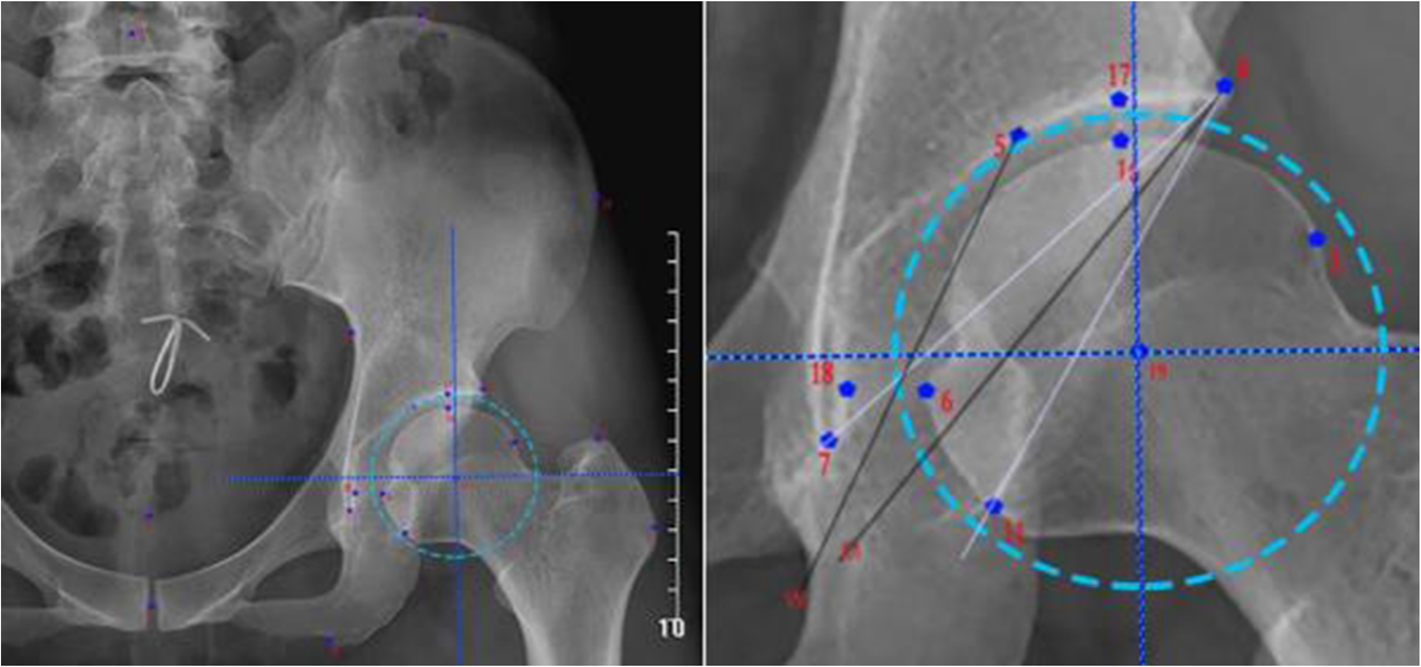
Identification of the anatomical landmarks on the AP radiograph to define the inner and outer edge of the weight-bearing area
